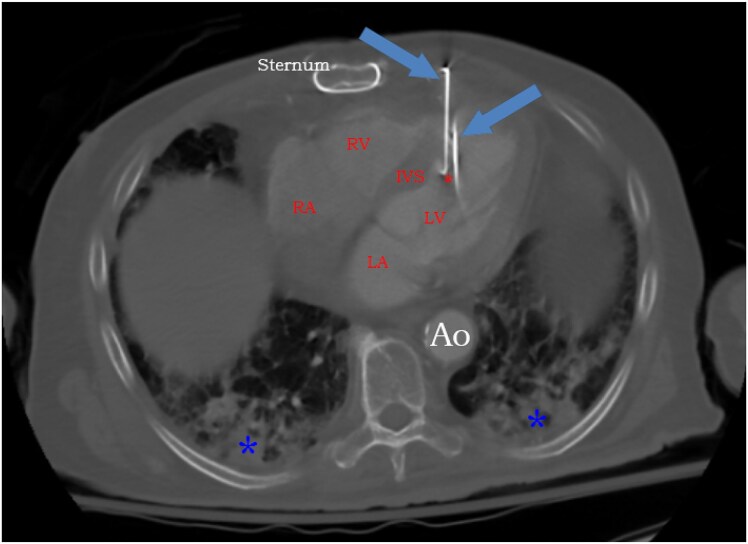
Computed tomographic angiography of the chest showing two nails penetrating through the heart. The tip of one of the nails (left blue arrow) terminates in the sub-endocardium of the mid interventricular septum (IVS). The tip of the other nail (right blue arrow) appears to terminate in the posteromedial papillary muscle (red asterisk). The third nail is not seen in this plane. Infiltrates are noted in the lower lobes of the lungs bilaterally (blue asterisks). Ao, descending thoracic aorta; LA, left atrium; LV, left ventricle; RA, right atrium; RV, right ventricle.
